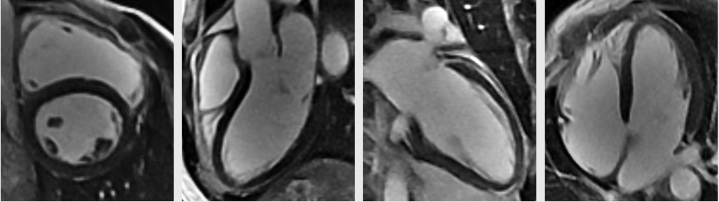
Cardiac Magnetic Resonance Imaging Absence of late gadolinium enhancement on cardiac magnetic resonance imaging.
